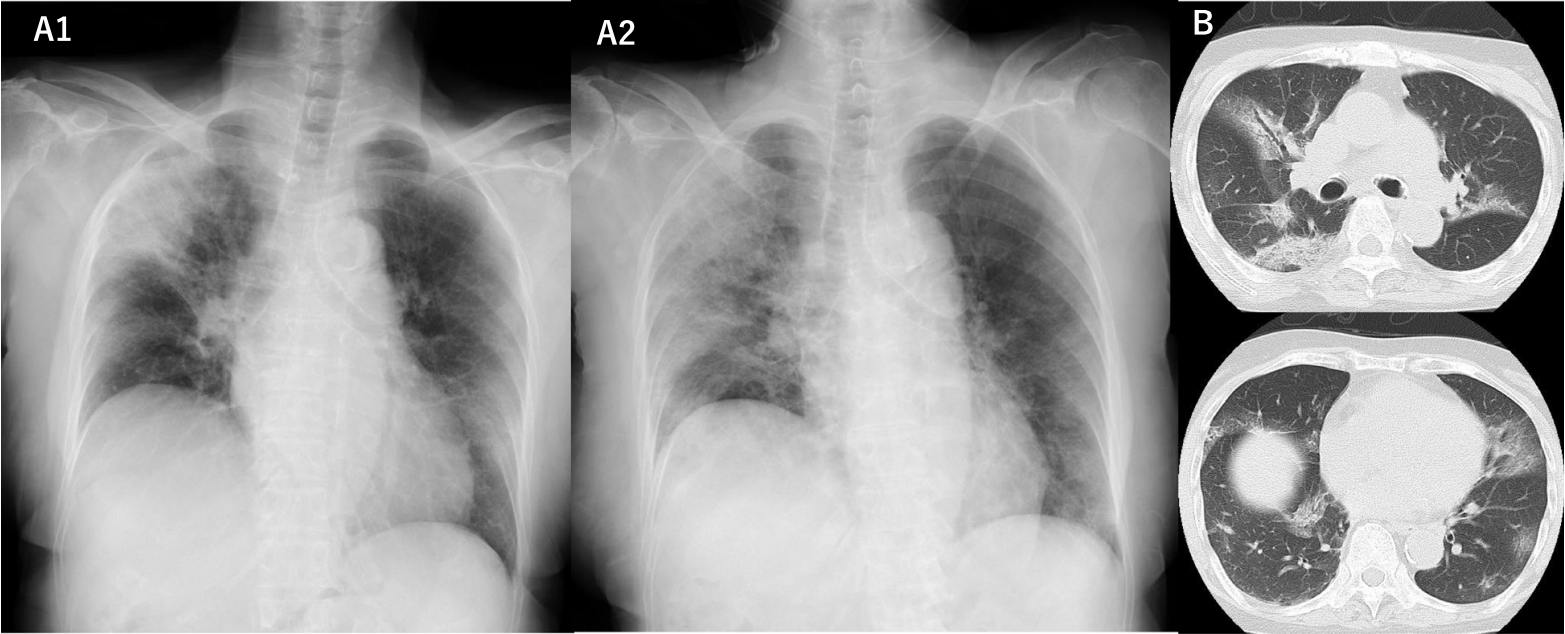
Axial images of HRCT and posteroanterior chest radiograph of patient 2. A, Chest radiograph obtained on hospital day 1 showed consolidation in a peripheral and mid zone distribution in right lung (A1). Follow up radiograph on hospital day 15 exhibited spread of opacities (A2). B, CT obtained on hospital day4, when she developed respiratory insufficiency, showing ground-glass shadow in both lungs
